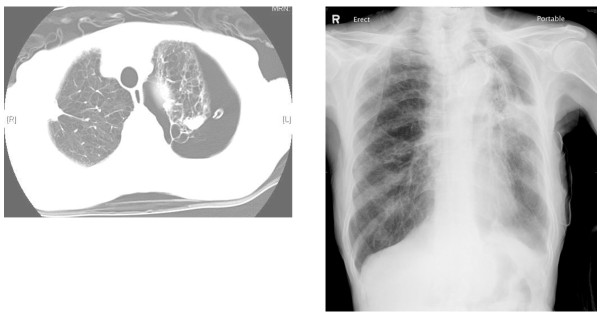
(a, *upper*) CT scan of patient #1 prior to EBV insertion showing extensive emphysematous changes and bullae in the underlying lung, a large left pneumothorax and the inserted chest tube. (b, *lower*) The airleak via the chest drain stopped immediately after EBV placements to occlude all upper lobe segments. The drain was removed. CXR on day 3 post-EBV insertion showed an incomplete expansion (but not total collapse) of the left upper lobe, which eventually re-expanded after bronchoscopic removal of the EBVs six weeks afterwards.
